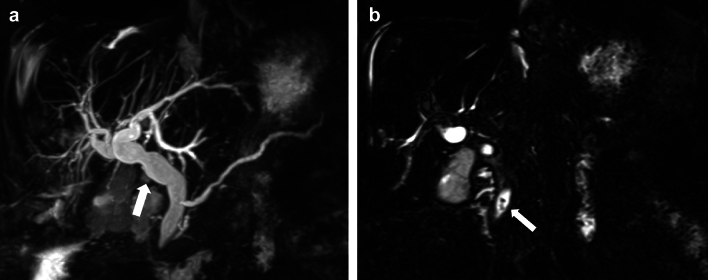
A 75-year-old female with retained gallstones post-cholecystectomy. MRCP abdomen was performed due to elevated liver function tests and an ultrasound of the abdomen showing worsening biliary dilatation. a and b Coronal MIP MRCP and Coronal 3D SPACE MRCP images show biliary dilatation (arrow in image a) and retained gallstones in the terminal common bile duct (arrow in image b)
